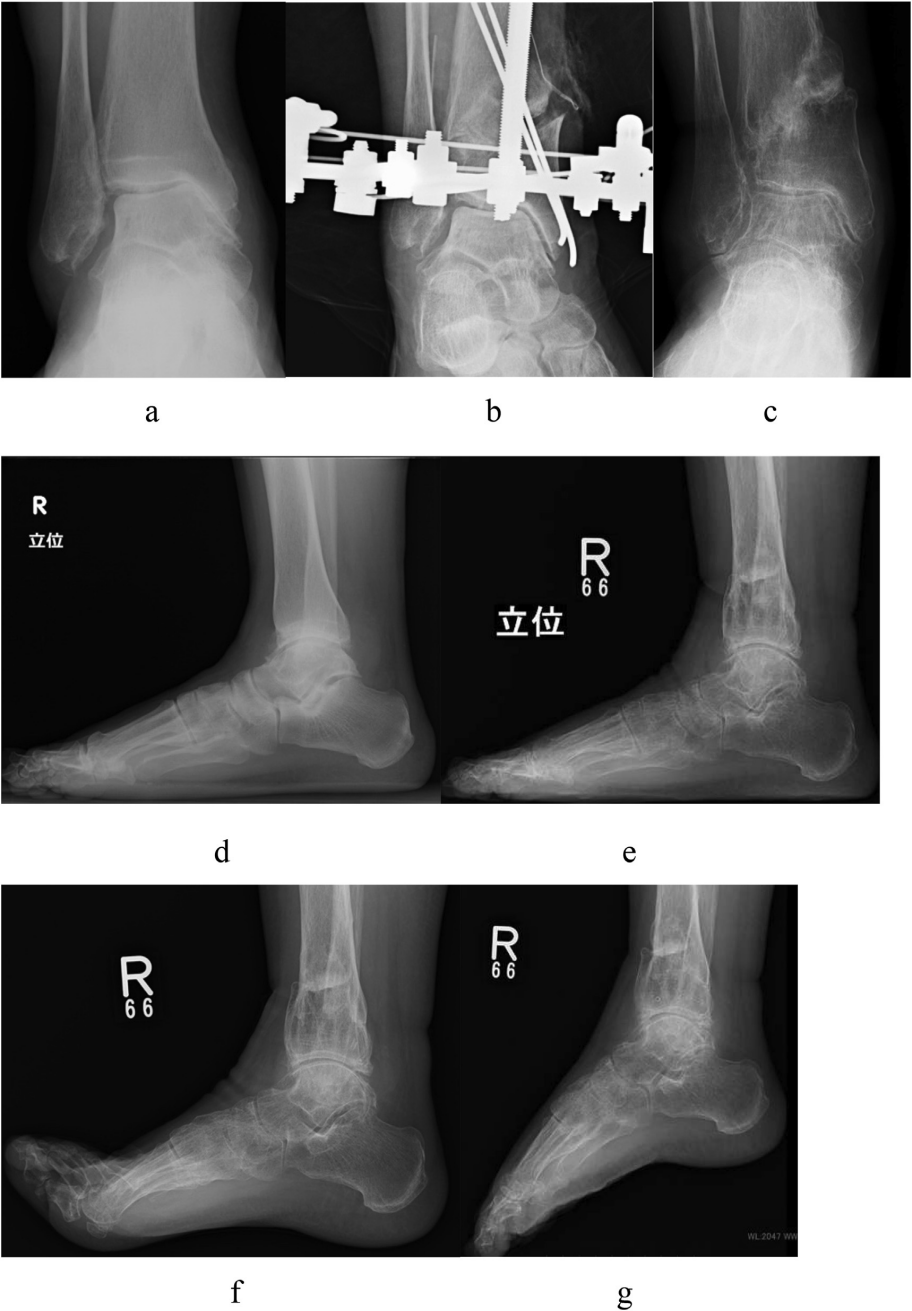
A 61-year-old female. Right ankle osteoarthritis, stage 3a (case 1). This is the typical stage 3a with talar medial shift and a large medial malleolar angle. a. Preoperative weight-bearing ankle radiograph (AP view). The medial joint space is narrowing and disappears partly. b. Ankle radiograph just after distal tibial oblique osteotomy (DTOO) (AP view). DTOO is performed and fixed using a circular external fixator. c. Weight-bearing ankle radiograph (AP view) at the last follow-up. The ankle joint congruency improves after DTOO. d. Preoperative weight-bearing ankle radiograph (lateral view). e. Postoperative weight-bearing ankle radiograph (lateral view). The talocrural joint space after DTOO is clearer than before DTOO. f. Postoperative ankle radiograph (dorsiflexion lateral view). g. Postoperative ankle radiograph (plantar flexion lateral view). The ankle range of motion is maintained after DTOO.
